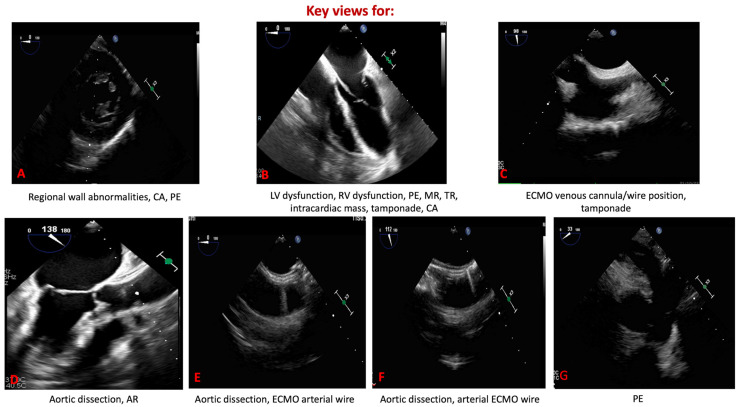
Key transesophageal echocardiographic views in emergency and critical care. (A) Trans-gastric mid-papillary short axis. (B) Mid-esophageal four-chamber view. (C) Mid-esophageal bicaval view. (D) Mid-esophageal aortic valve axis view. (E) Mid-esophageal short-axis aortic view. (F) Mid-esophageal aortic long axis view. (G) Mid-esophageal short-axis view of the ascending aorta. CA: cardiac arrest; PE: pulmonary embolism; LV: left ventricle; RV: right ventricle; MR: mitral regurgitation; TR: tricuspid regurgitation; ECMO: extracorporeal membrane oxygenation; AR: aortic regurgitation.
